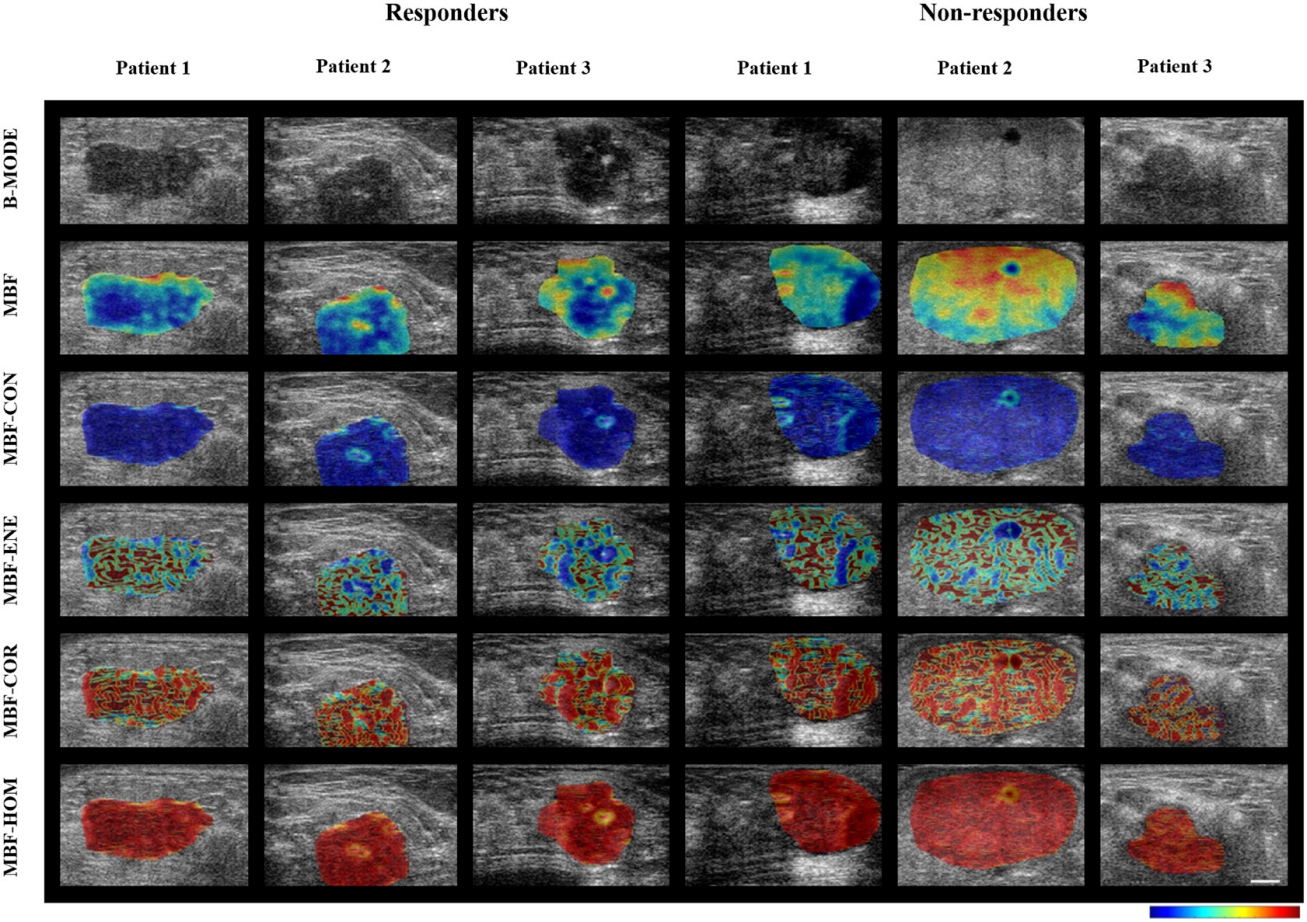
Parametric maps for the two response groups. Representative ultrasound B-mode images with MBF parameter overlays, and MBF-CON, MBF-ENE, MBF-COR, and MBF-HOM feature overlays acquired from three responder and non-responder patients before chemotherapy. To generate the parametric maps, the values were normalized across all the patients (irrespective of treatment response) for individual features. The normalized values for the feature were then represented quantitatively as colors across the sub-regions of interest across the entire tumor volume. The scale bar in ultrasound images represents 5 mm. The color bars represent scales for the MBF parameter of -9 to 18 dBr, for the MBF-CON parameter of 0 to 2.7, for MBF-ENE parameter of 0 to 1, for the MBF-COR parameter of -0.4 to 0.9, and for MBF-HOM parameter of 0 to 1. MBF: Mid-band fit; CON: Contrast; ENE: Energy; COR: Correlation; HOM: Homogeneity.
